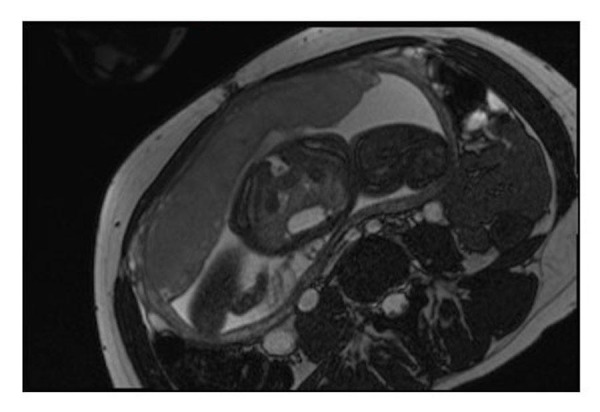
Meningocele An axial T2-weighted image demonstrating a meningocele.
